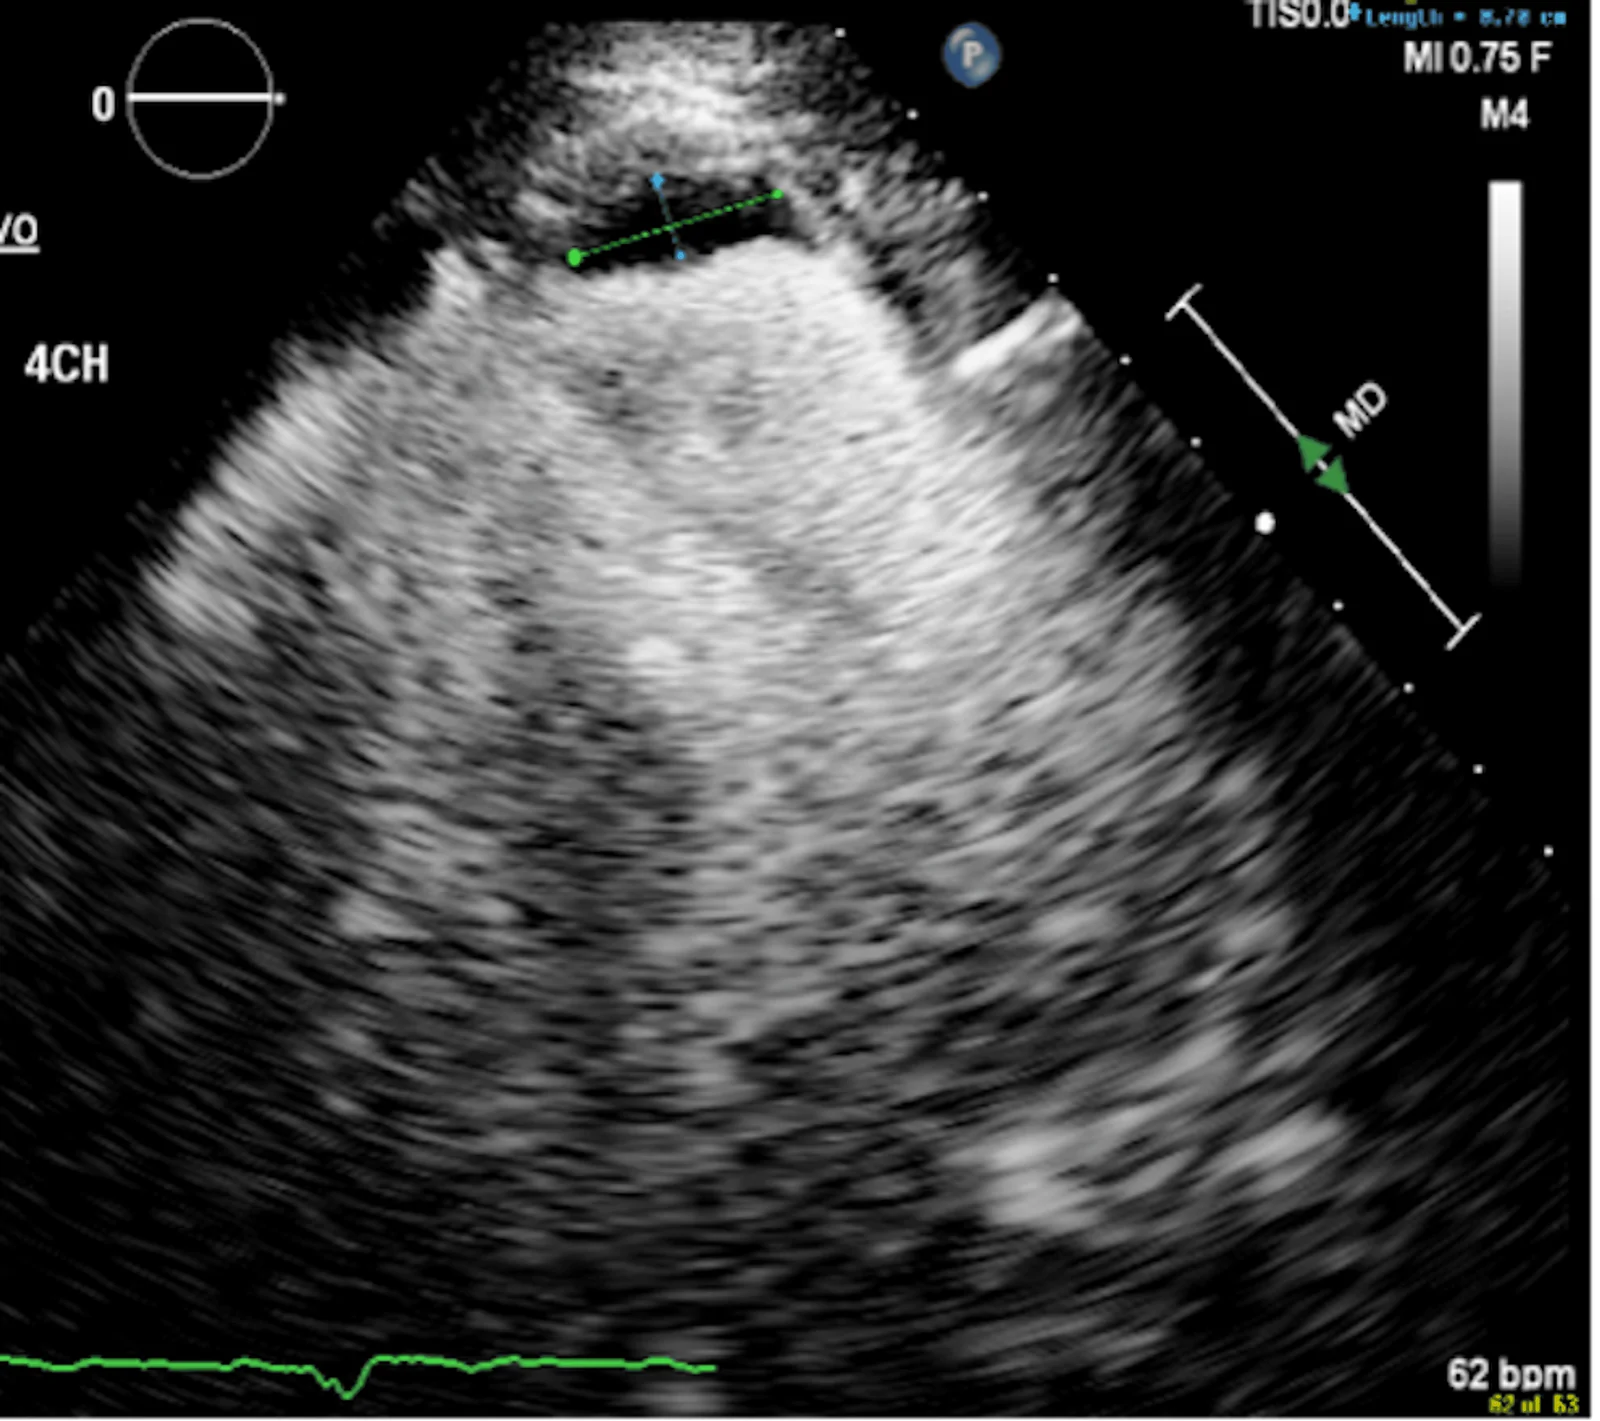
Repeat TTE in April 2026 with contrast agent (Definity) (A4C view) Apical thrombus still present TTE: transthoracic echocardiogram, A4C: apical four-chamber, LV: left ventricle
